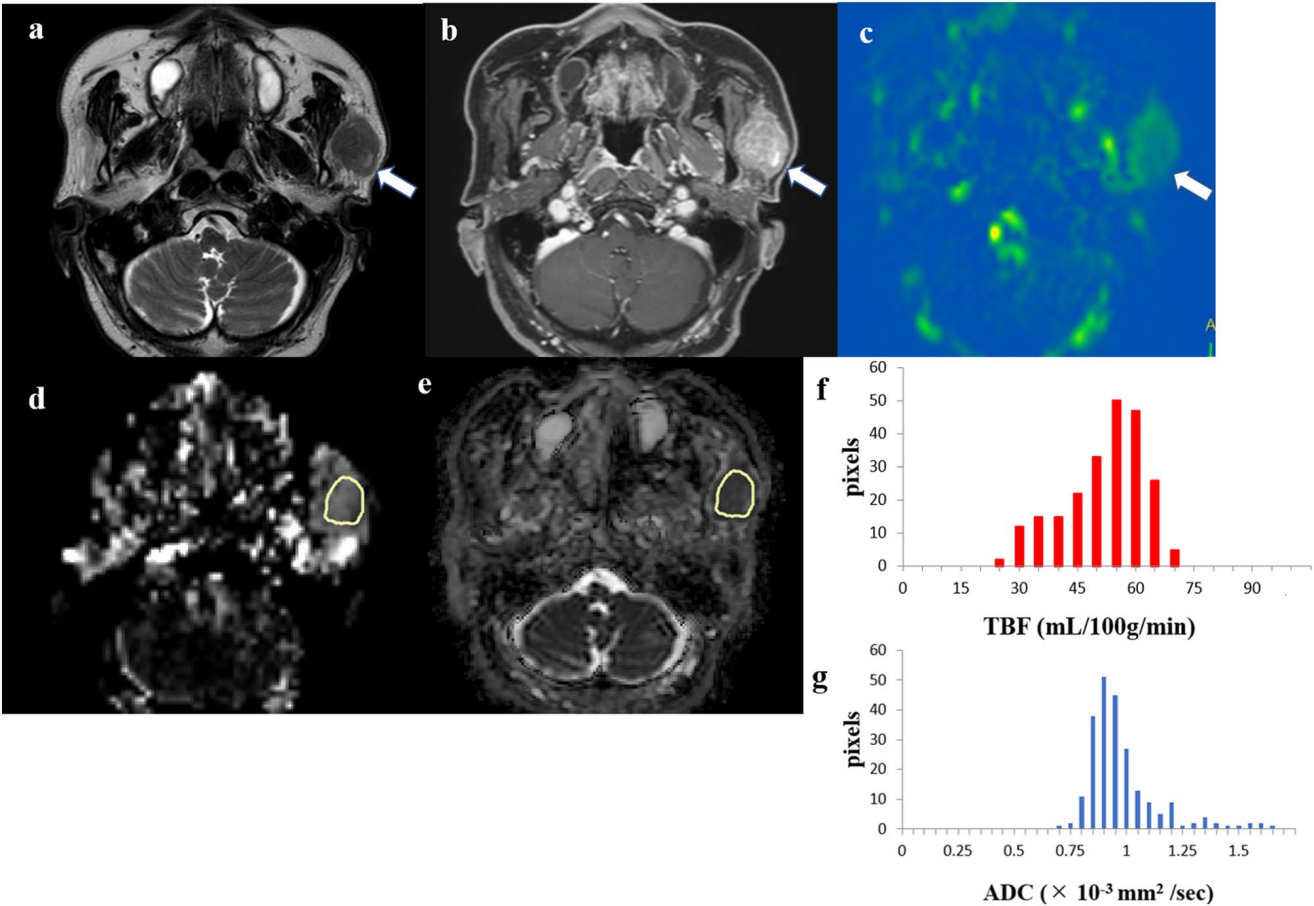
An 80-year-old male patient with a carcinoma ex pleomorphic adenoma in the left parotid gland. T2-weighted image (a) showing an iso signal intensity lesion (*arrow*). Contrast-enhanced 3D-T1-weighted image (b) showing homogeneous contrast enhancement (*arrow*). Tumor blood flow (TBF) color map (c) showing medium TBF (*arrow*). The region of interest (ROI) was manually drawn on the apparent diffusion coefficient (ADC) map of the software (e, *yellow*), and the ROI was copied from the ADC map to the TBF map of the software (d, *yellow*). The TBF histogram (f) and ADC histogram (g) are presented. The 50th percentile of the TBF value was 50.92 mL/100 g/min, whereas the 10th percentile of the ADC value was 0.82 × 10^−3^ mm^2^/s.
